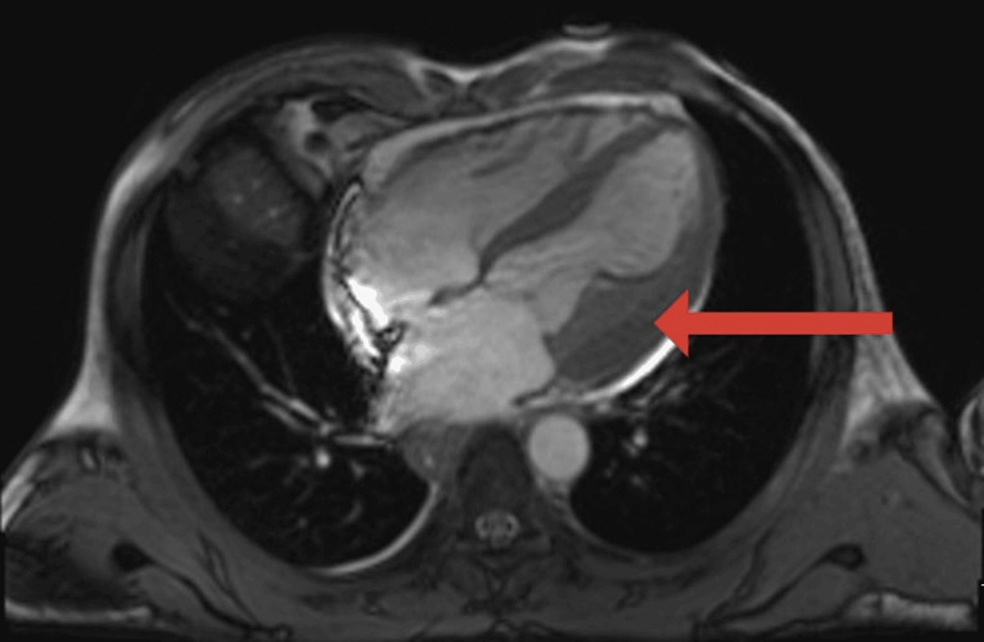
Cardiac MRI showing features of the focal area of Loffler’s endomyocarditis in the anterolateral wall of the basal and mid ventricle with associated intracardiac thrombus formation
